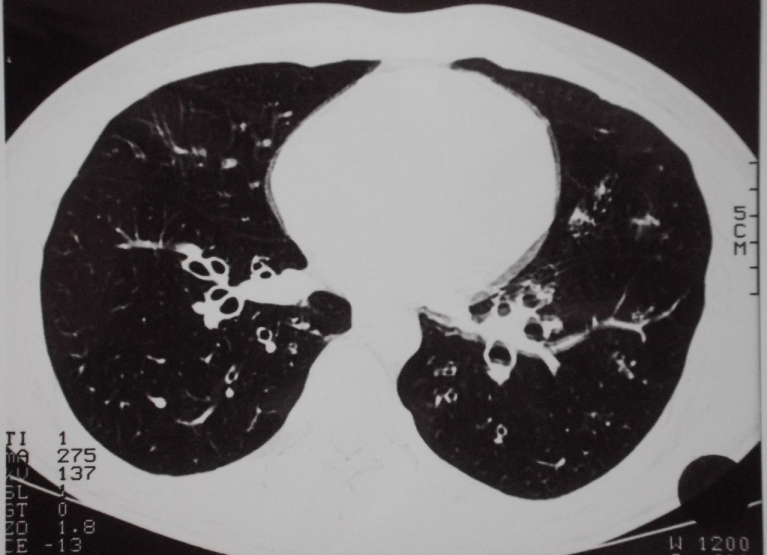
Thorax CT shows cylindrical bronchiectasis and thick bronchial walls. Lung parenchyma is mostly unchanged. (Department of Radiology of Philipps-University Marburg)
